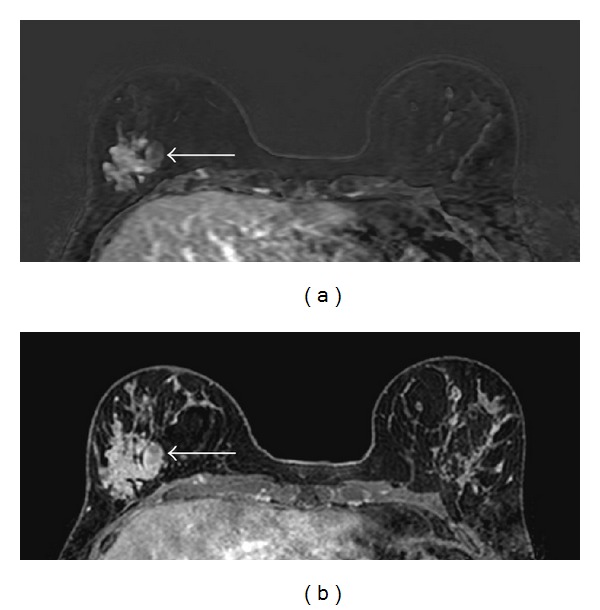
Contrast-enhanced (subtraction) MR images (a) and contrast-enhanced T1-weighted MR images (b) show a large, lobulated heterogeneously enhancing mass with irregular borders in the lower lateral quadrant of the right breast (arrows).
